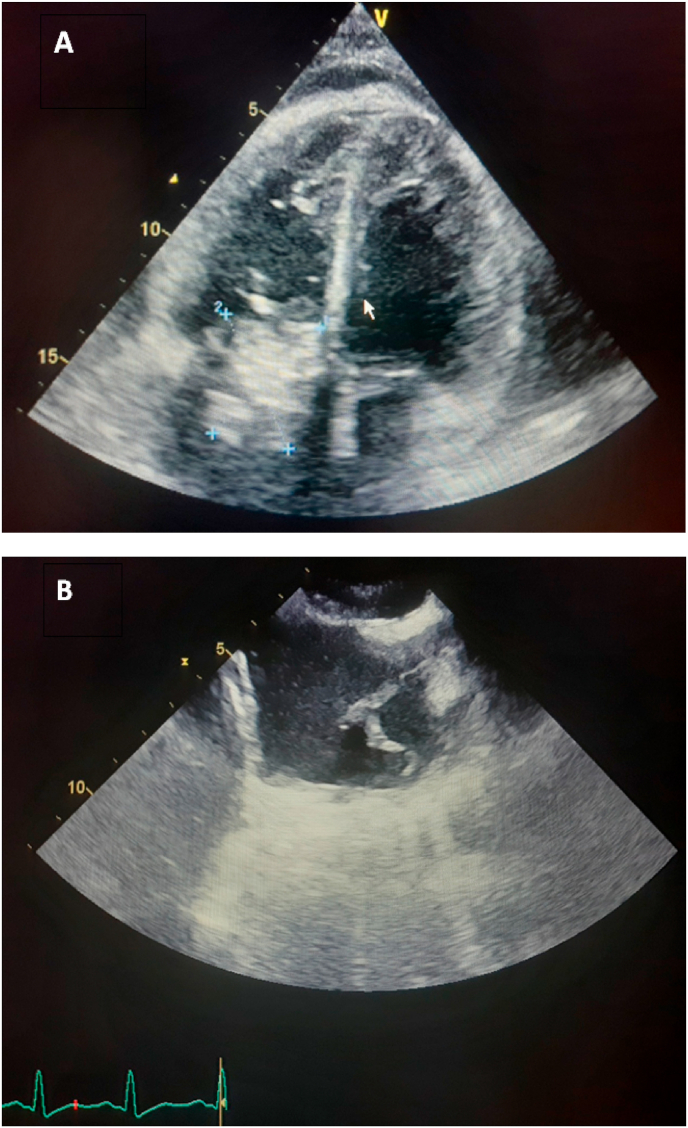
Cases of right-sidded infective endocarditis (A). transthoracic apical 4 chamber view showing vegetation attached to the tricuspid valve. (B) Transesophageal echocardiography image showing vegetation located on the lead of a port catheter.
